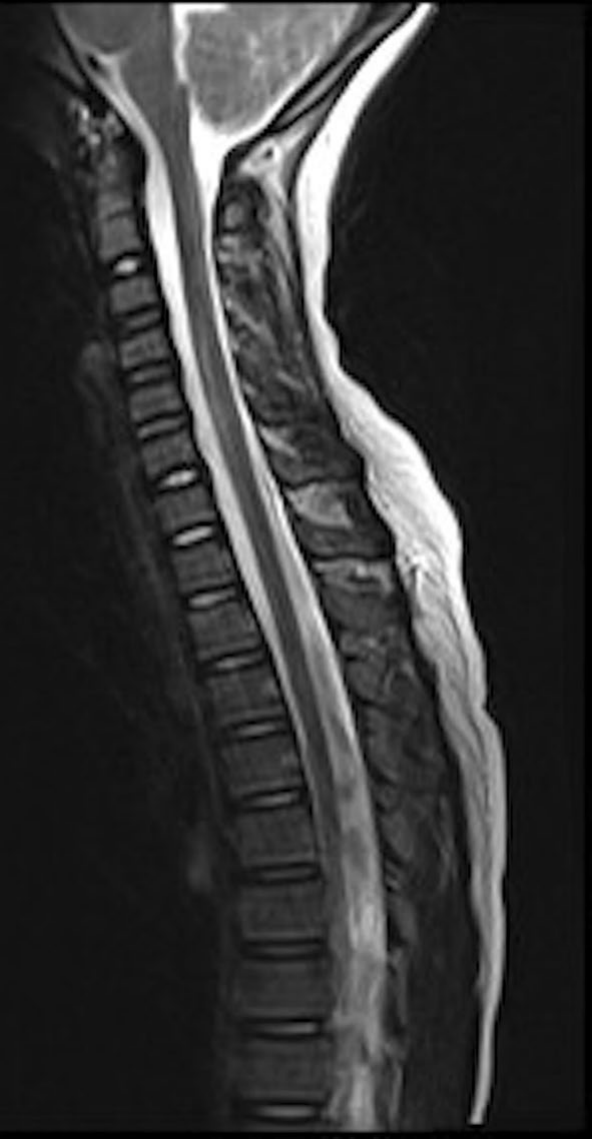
Sagittal T2-weighted MR image showing SCIWORA. Note the hyperintensity of cord oedema following trauma to the cervical spine
